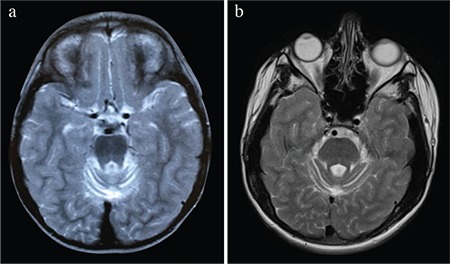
Compared to previous magnetic resonance imaging, the atrophy of the cerebellum progressed; (a) performed when he was of 3 years and (b) performed 12 years after the initial magnetic resonance imaging.
